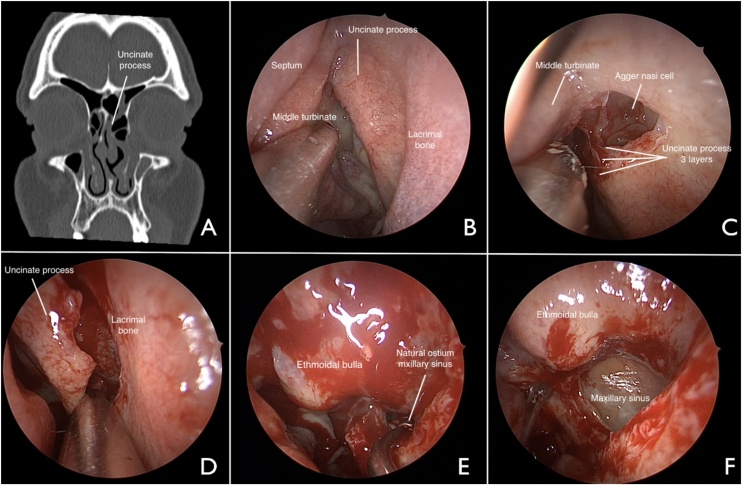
Uncinectomy using the agger nasi technique in a patient with chronic rhinosinusitis. A: computed tomography of paranasal sinuses (bone window, coronal slice). Uncinate process visible on the left side. B-F: endonasal approach to the left nasal fossa (45-degree scope view). B: exposure of the middle meatus. C: horizontal incision with shaver. C: identification of the 3 layers of the uncinate process (medial mucosa, bone, lateral mucosa). D: detachment of the uncinate process from the lacrimal bone anteriorly and inferiorly. E: identification of the natural drainage ostium of the maxillary sinus. F: final appearance of maxillary antrostomy after complete resection of the uncinate process.
